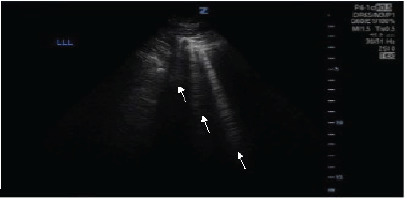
Initial chest radiograph upon emergency department arrival (left) and 15 hours after presentation (right) of a patient with chloramine exposure.
